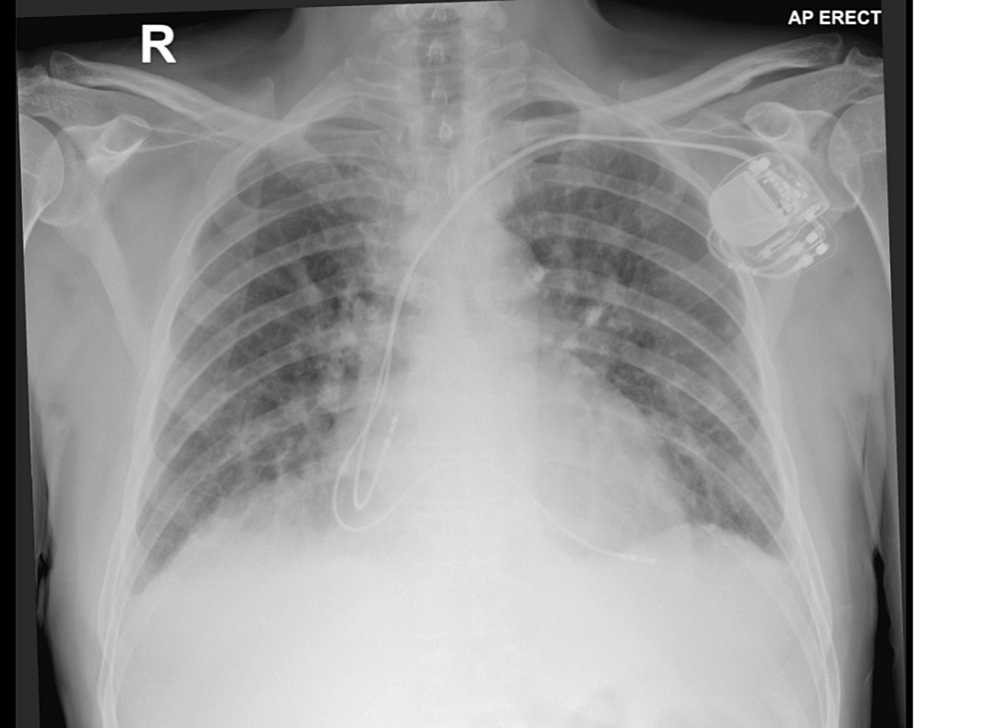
CXR AP view showing the lead in the RA and lead in the LV which is hard to differentiate from the normally positioned RV lead CXR: Chest X-ray; AP: antero-posterior; RA: right atrium; LV: left ventricle; RV: right ventricle
